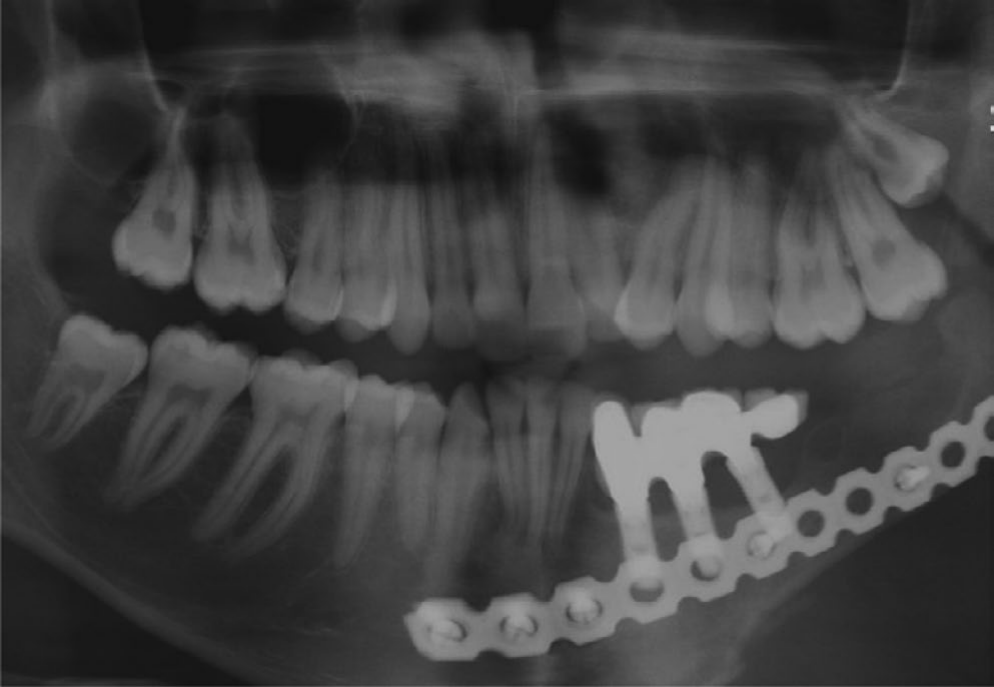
Radiograph after 3 years follow‐up
